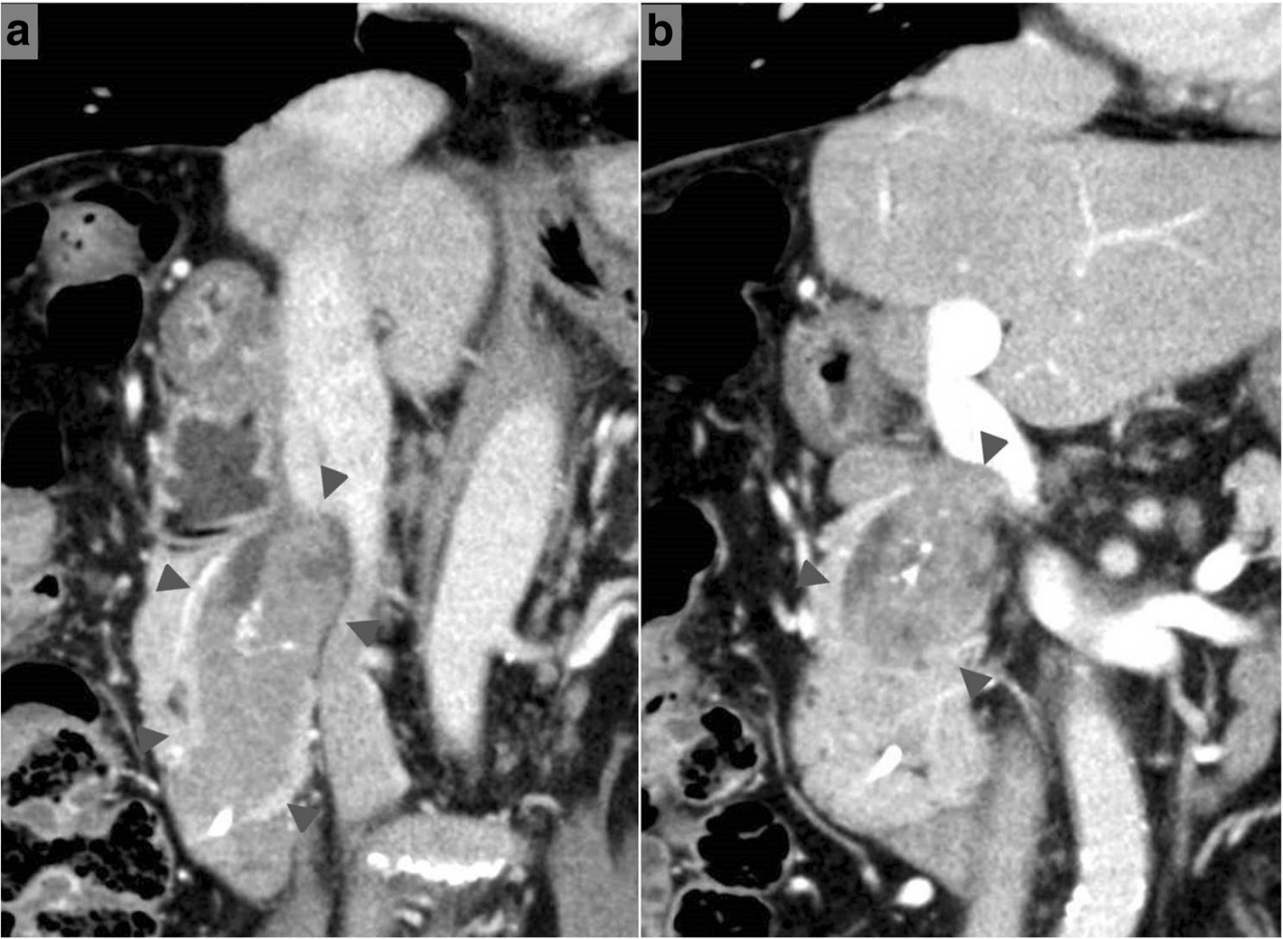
Computed tomography images obtained in February 2014. An obvious mass with clustered calcifications (*arrowheads*) in the dilated intrapancreatic bile duct (a) dorsal side (b) ventral side
